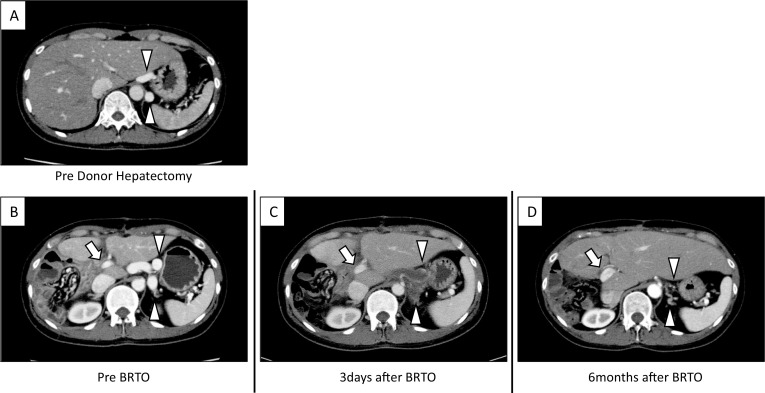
Contrast-enhanced computed tomography before and after B-RTO. A Preoperative contrast-enhanced computed tomography revealed a congenital portosystemic shunt 9 mm in diameter (arrowheads) originating from the left gastric vein (LGV) and draining to the left renal vein (LRV). B–D After donor hepatectomy, the portal trunk narrowed and the congenital portosystemic shunt widened 12 mm in diameter. After the B-RTO, the portal trunk expanded (arrows), and liver regeneration accelerated after balloon-occluded retrograde transvenous obliteration (B-RTO). Embolization of the LGV–LRV was successfully achieved and became inconspicuous 6 months after B-RTO (arrowheads)
